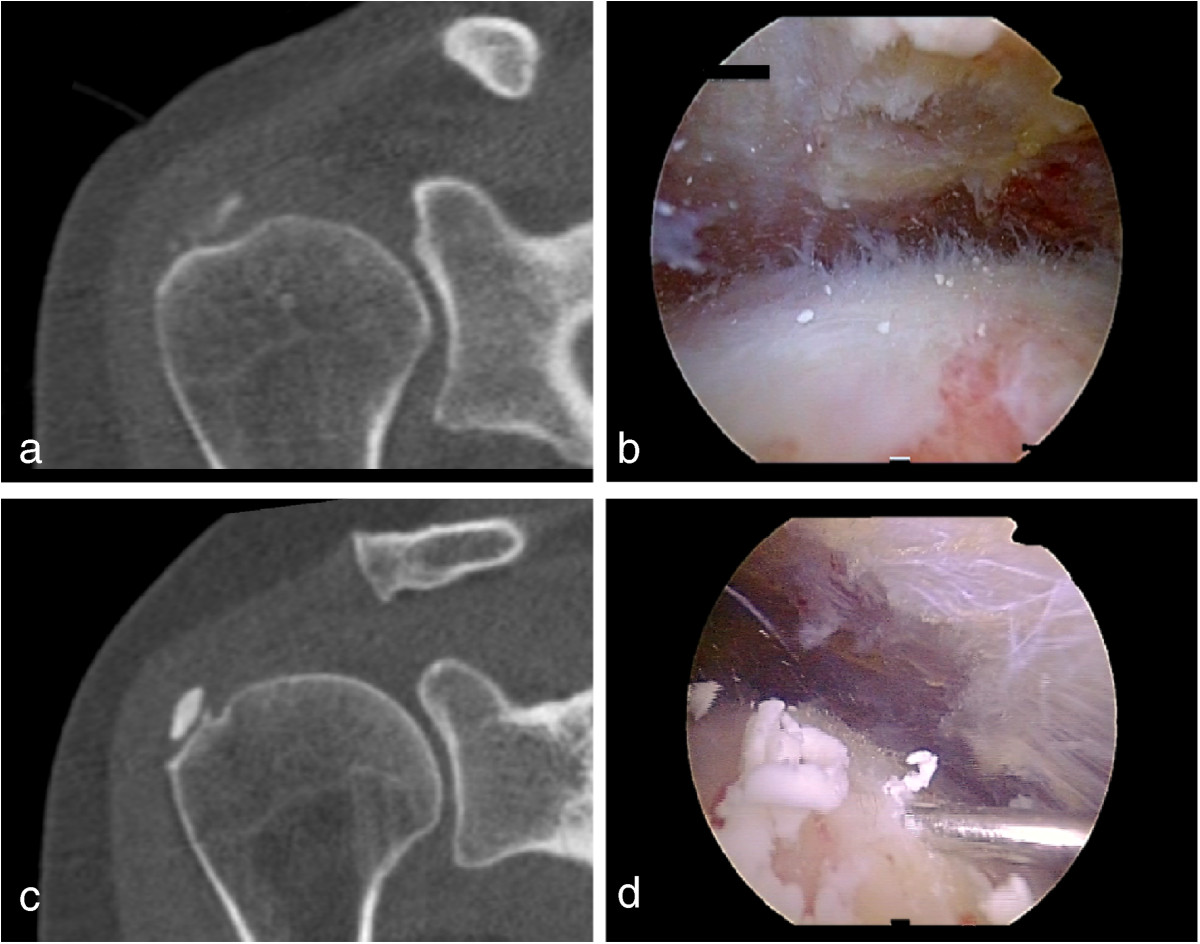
CT scans with corresponding intraoperative findings. In the left row CT scans of a patient with a liquid-soft deposit (a) and with a viscous-solid deposit (c) are displayed. In the right row the corresponding intraoperative findings are displayed (liquid-soft deposit (b), viscous-solid deposit (d).
